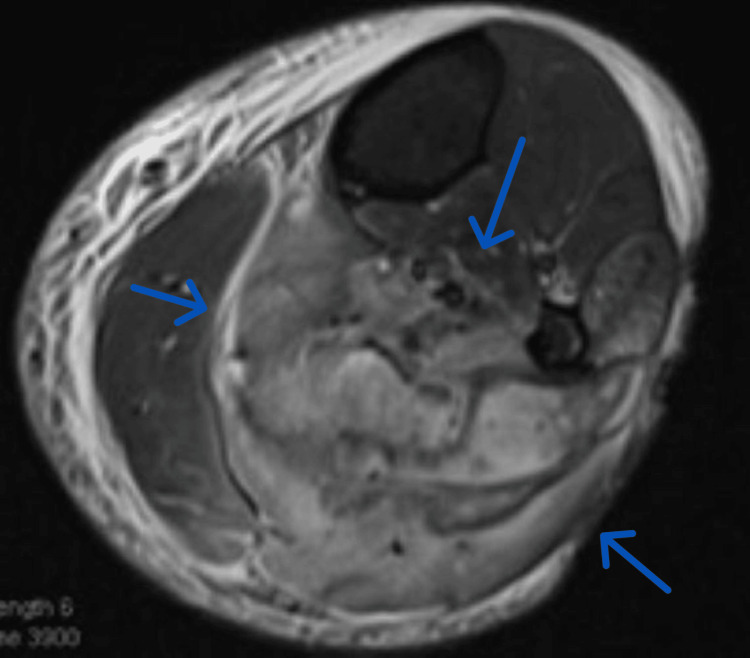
Left lower extremity MRI with abnormal posterior compartment signal.
